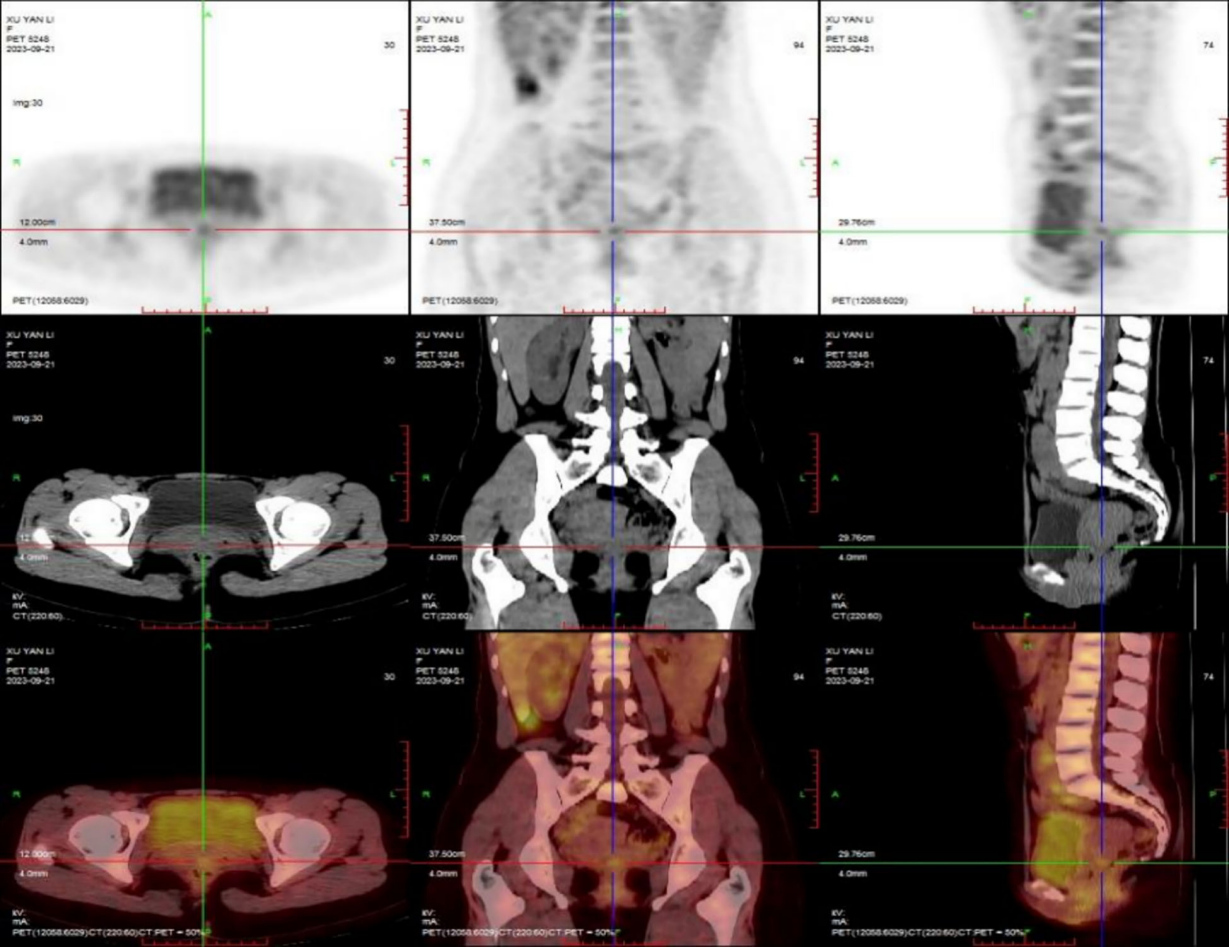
PET-CT imaging shows slightly thickened soft tissue in the cervix and increased FDG metabolism. The “cross” intersection in the figure is the lesion site.
